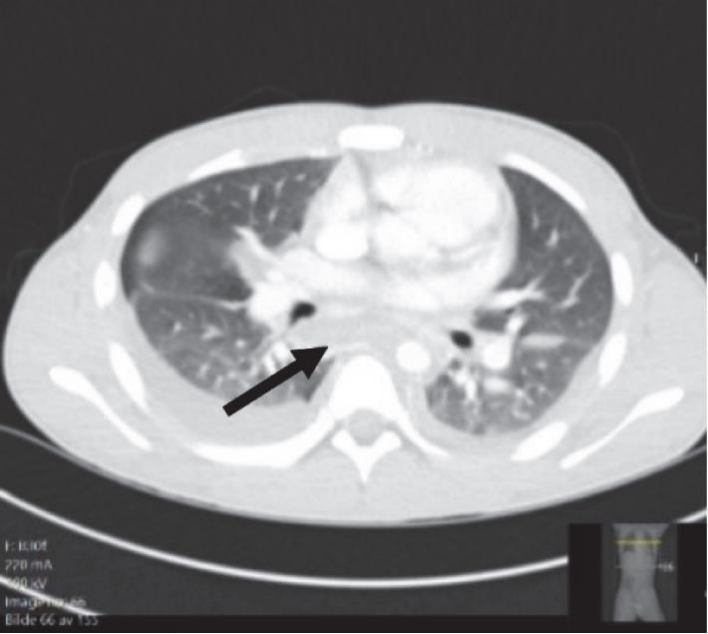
CT performed upon admission; arrow indicates increased soft tissue in the mediastinum.
